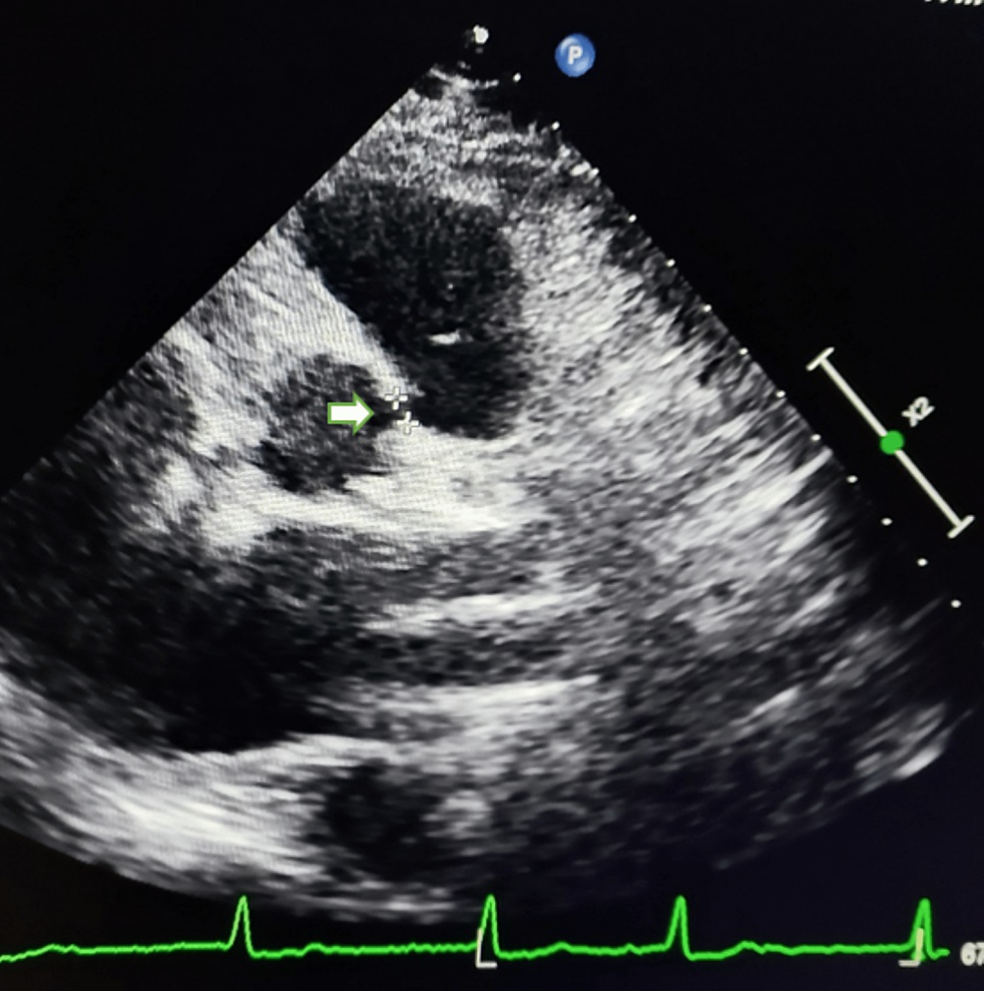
Bi-dimensional echocardiography, on short axis view, demonstrating the small size of the aortopulmonary window (white arrow), above the pulmonary valve
